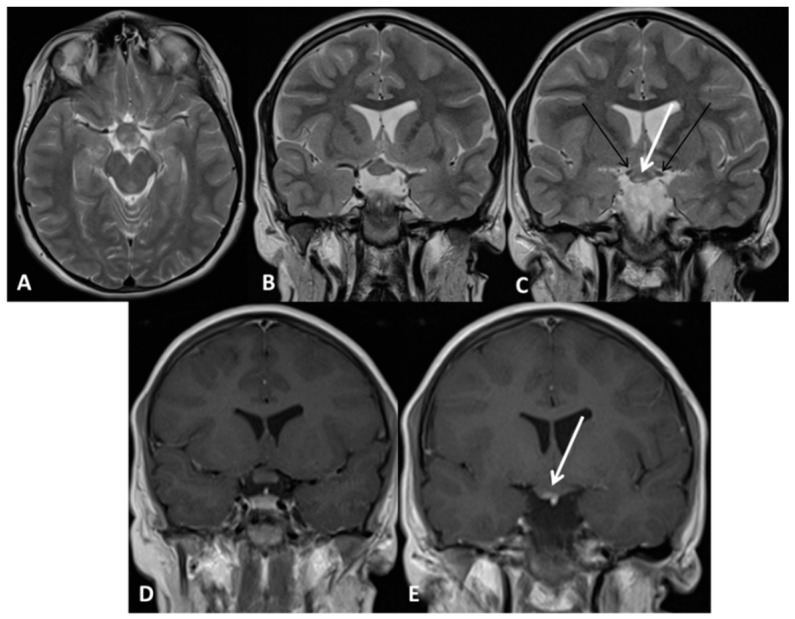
Chiasm and hypothalamic glioma 2aH+ (Subject 09 OPG). Brain MRI. (A) Axial T2 weighted images (w.i); (B,C) Coronal T2 w.i. (B,C); Panel A, B and C show enlargement of the chiasm and hypothalamus with a small hyperintense area (white arrow in C), while retrochiasmatic tracts are spared (black arrows in C). (D,E) Coronal T1 w.i. after gadolinium administration showing enhancement in the hyperintense corresponding area (arrow in E). OPG: Optical pathways glioma; MRI: Magnetic resonance imaging.
